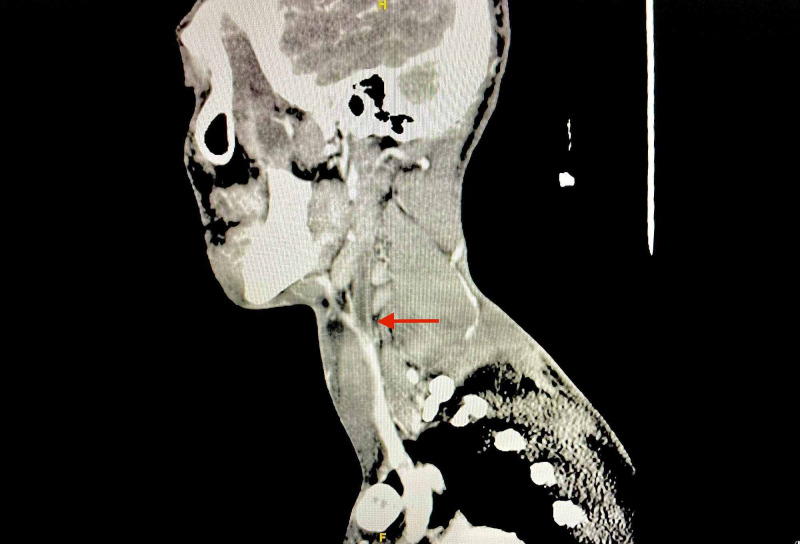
CT neck with contrast (sagittal view) showing filling defect of the left internal jugular vein (red arrow). CT, computed tomography
